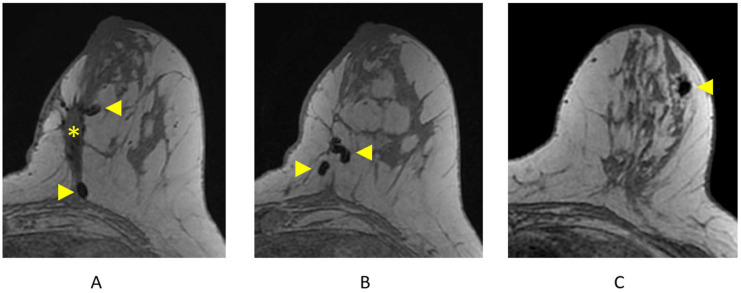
Surgical and biopsy clips on axial T1-weighted breast MRI. (A) Lumpectomy site (asterisk) and areas of focal susceptibility representing surgical clips (arrowheads); (B) additional surgical clips (arrowheads); (C) focal susceptibility artifact representing a breast biopsy clip/tissue marker (arrowhead).
